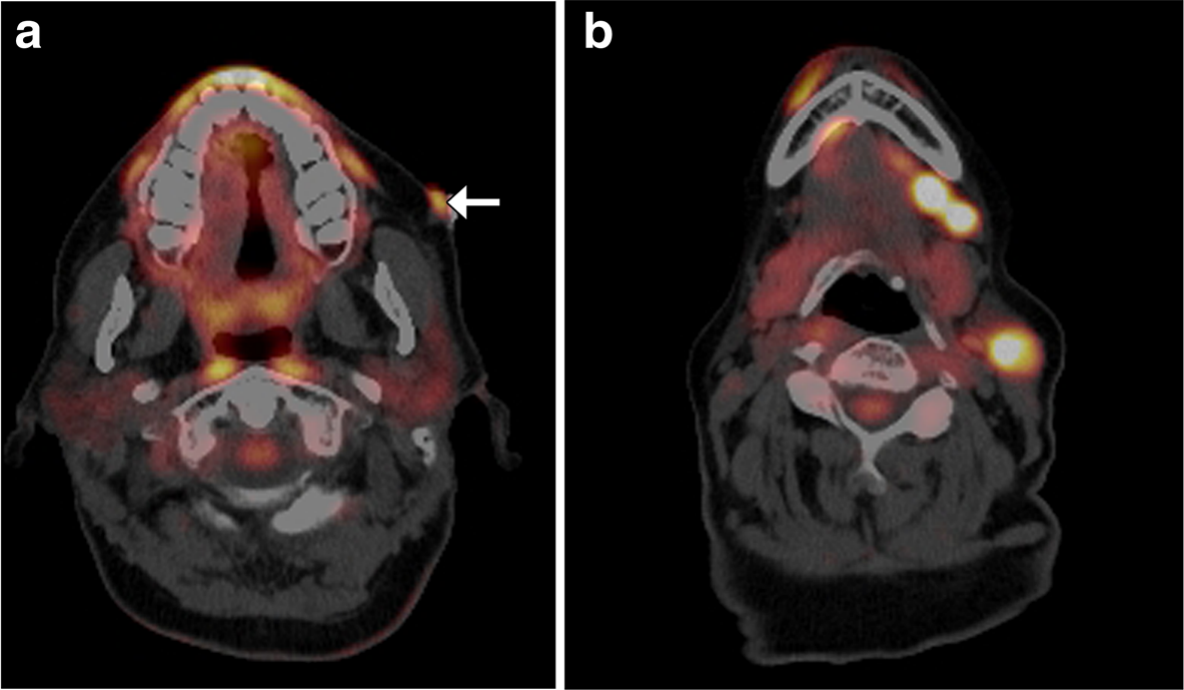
Merkel cell carcinoma. Axial fused ^18^FDG-PET/CT images (a and b) show a hypermetabolic left cheek lesion (arrow) and ipsilateral suprahyoid metastatic lymphadenopathy
